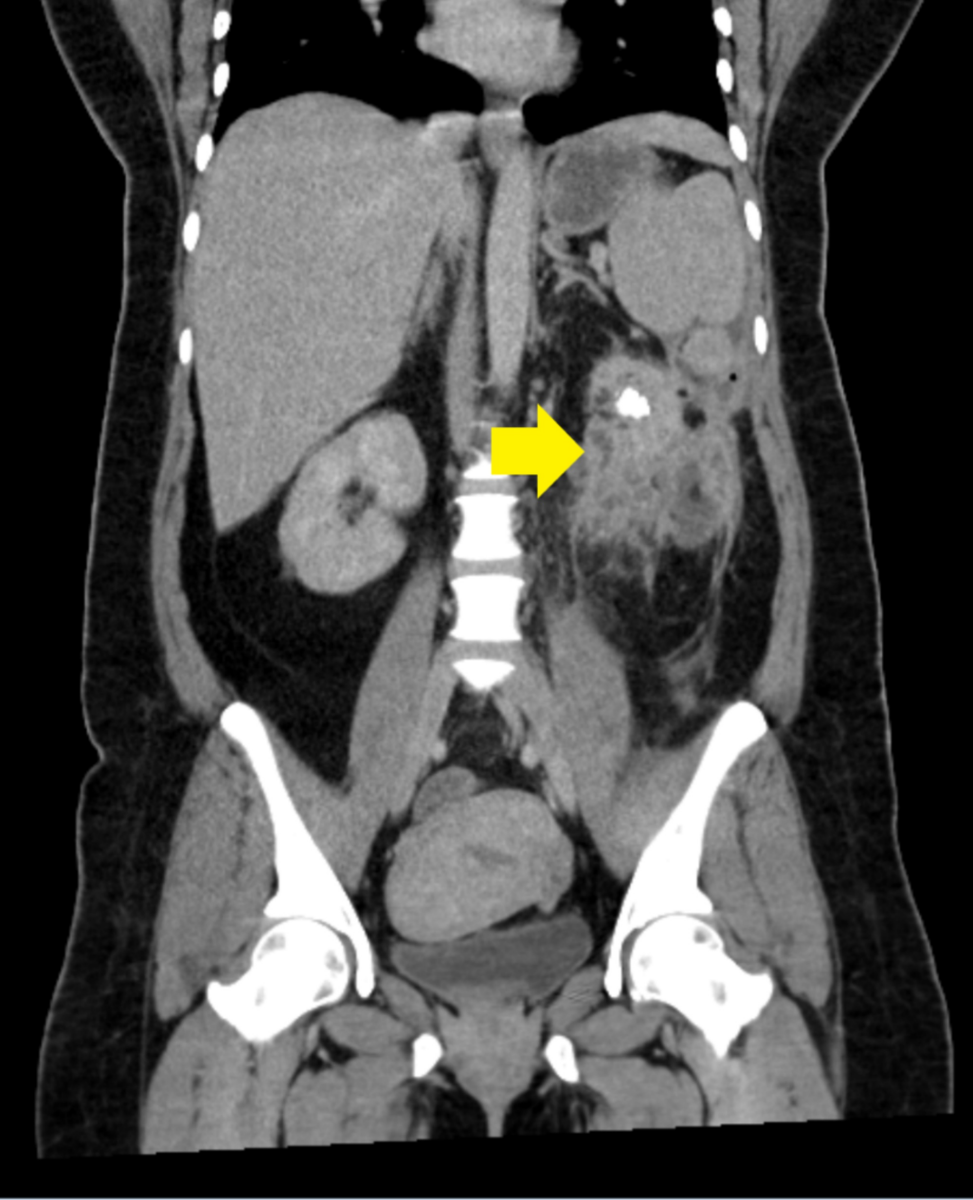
Computed tomography scan of the abdomen and pelvis showing xanthogranulomatous pyelonephritis
